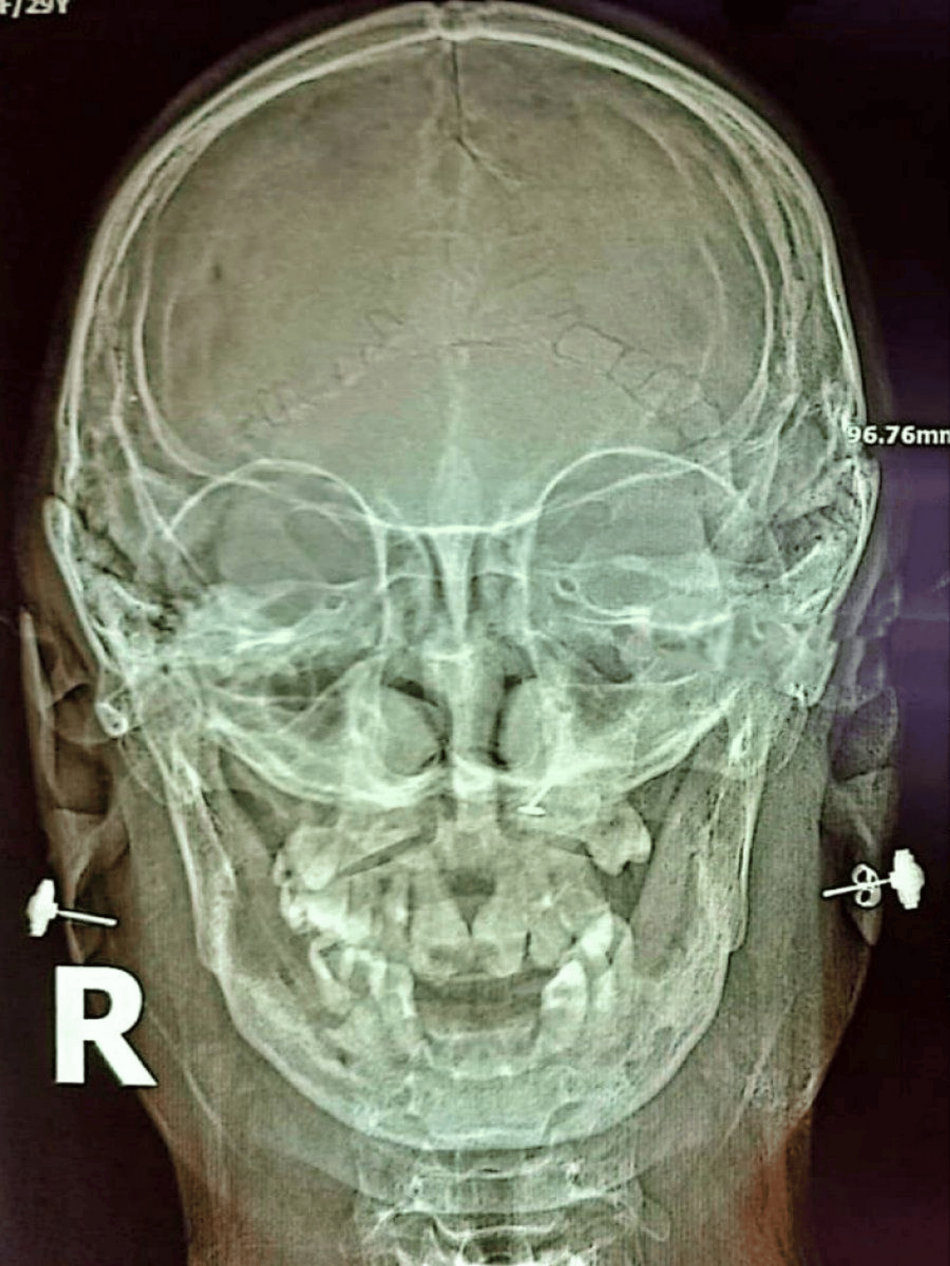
Wormian bones, absence of frontal sinus, and maxillary sinus hypoplasia were noted in the skull X-ray.
